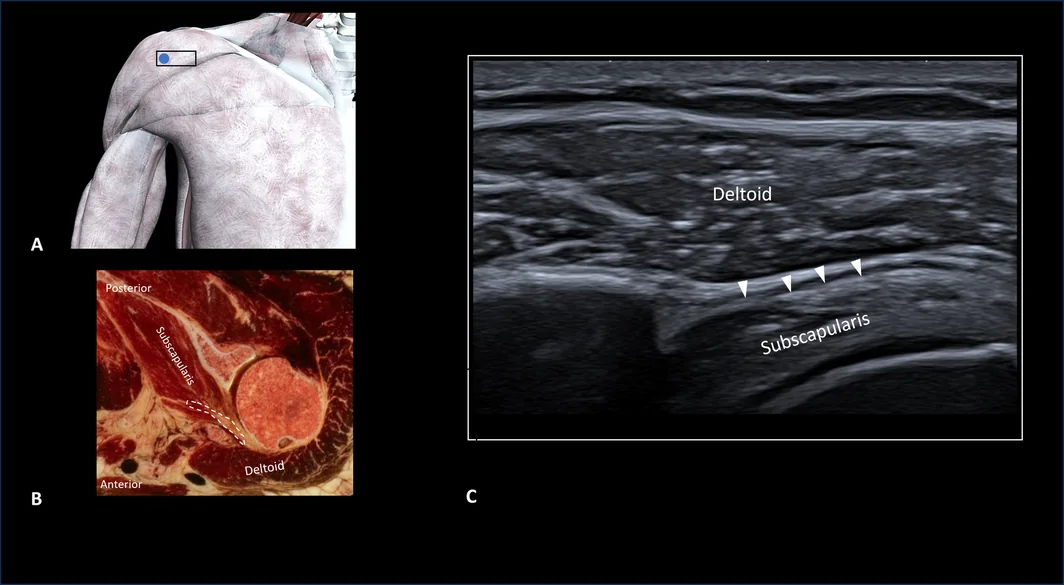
Subscapularis fascia. A, Probe positioning. B, Coronal anatomical section derived from the Visible Human Project®. *Courtesy of the U.S. National Library of Medicine (NLM), National Institutes of Health* (dotted line: subscpularis fascia). C, Ultrasound image revealing the subscapularis fascia (arrowheads).
